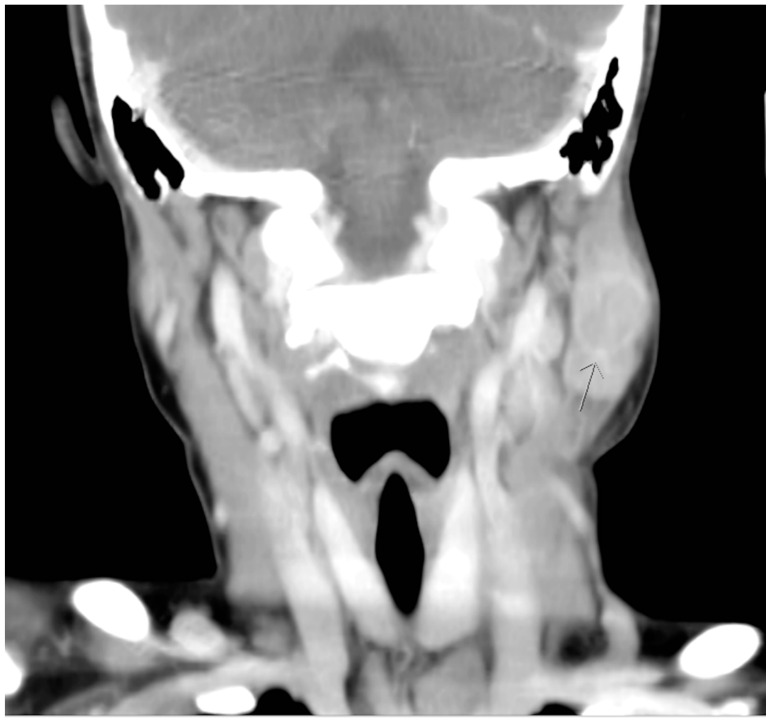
Coronal computed tomographic view revealing a hypodense cyst with ill-defined margin in the left parotid gland. (arrow head).
